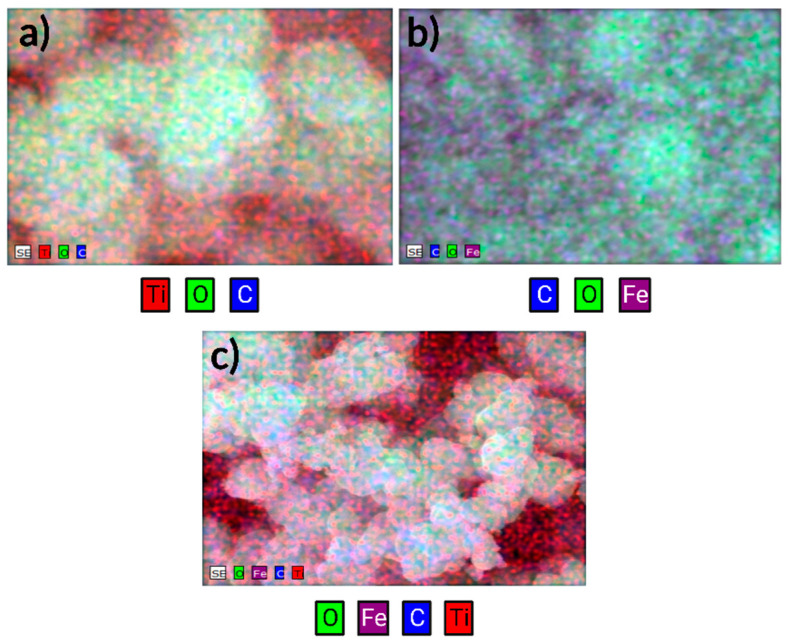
Energy dispersive X-ray elemental analysis of (a) TiO_2_, (b) Fe_3_O_4_, and (c) TiO_2_/Fe_3_O_4_.
